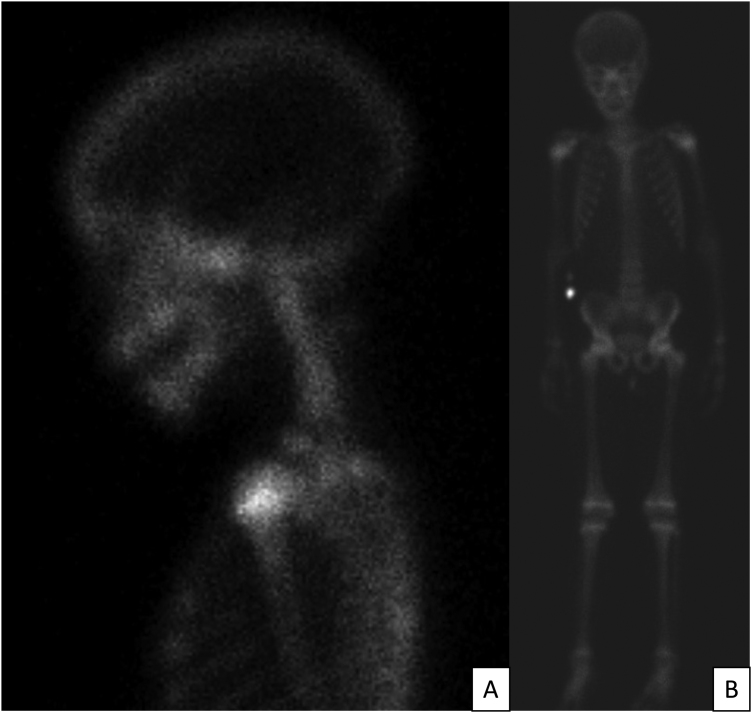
(A) detail in neck region; (B) Whole body bone scintigraphy showing normal distribution of the radiopharmaceutical, according to age and bone framework.
